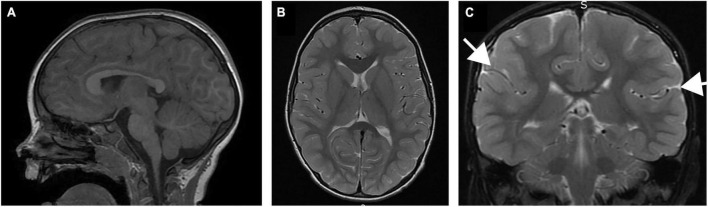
Brain MRI of patient performed at 22 months of age. (A) Sagittal T1 image demonstrating normal size and morphology of the corpus callosum, cerebellum and brainstem. (B) Coronal T2 image demonstrating normal configuration of the basal ganglia, including fiber organization of the internal capsule. (C) Coronal T2 image demonstrating subtle thickening in the sylvian fissure (arrows).
